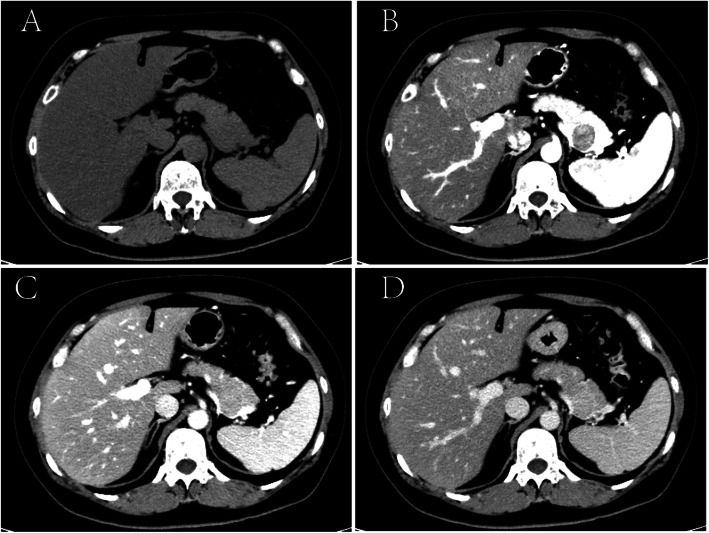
CT scan of abdomen showing a round low-density mass in the tail of pancreas with heterogeneous enhancement. a plain scan, b arterial phase, c venous phase, d delayed phase
